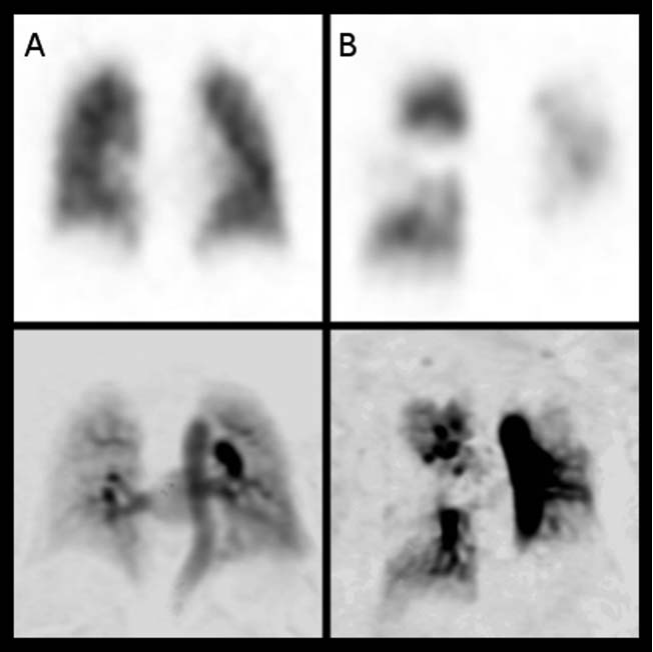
Matched slices from 3D coronal SPECT perfusion images (top row) and DCE MR perfusion images (bottom) in a patient with normal lung perfusion (A) and with CTEPH (B). This shows the typical wedge‐shaped perfusion defects (arrows) in the right mid, left lower, and left upper zones on the MR and the SPECT imaging of patient B. Note the images are presented on an inverse gray scale as reviewed clinically.
